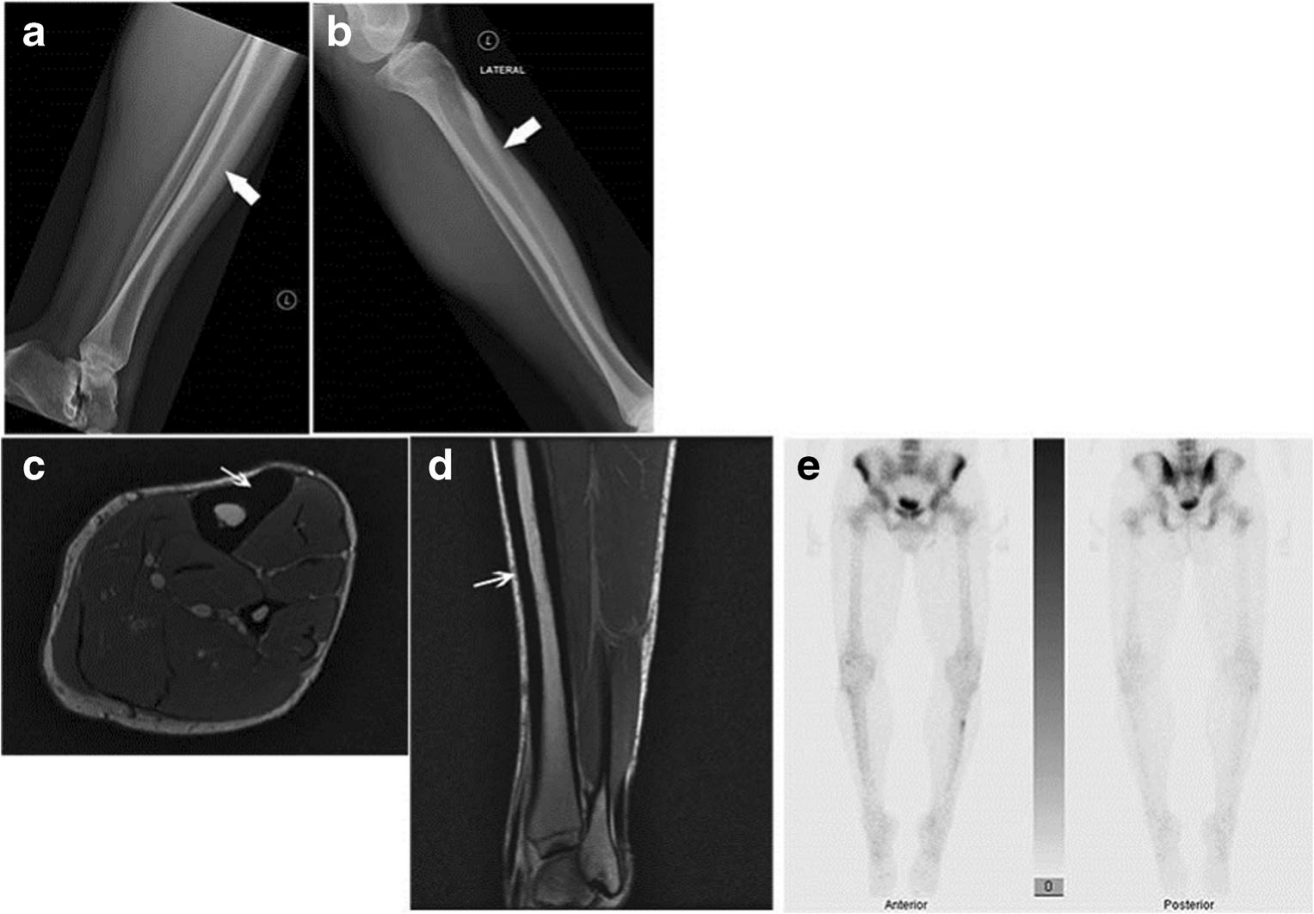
Ribbing disease. Lateral radiograph of right tibia a shows smooth thickening along the anterior cortex; this radiograph was obtained when the patient was 19 years of age. 7 years later, a follow-up lateral radiograph obtained as a young adult b shows further increased thickening along the anterior tibial cortex with narrowing of the medullary cavity. Axial c and sagittal d PD MR images of the tibia obtained at the same time as the second radiograph reveal smooth cortical thickening anteriorly. No unusual abnormal uptake of radiotracer was noted on the concomitantly performed Tc-99m MDP labeled bone scan eKenny–Caffey syndrome (KCS):Kenny–Caffey syndrome (KCS) is a very rare dysmorphologic syndrome. The hallmark of this syndrome is a proportionately short stature, cortical thickening and medullary cavity obliteration/narrowing of tubular bones, delayed closure of anterior fontanelle, eye abnormalities, and hypoparathyroidism [5].
